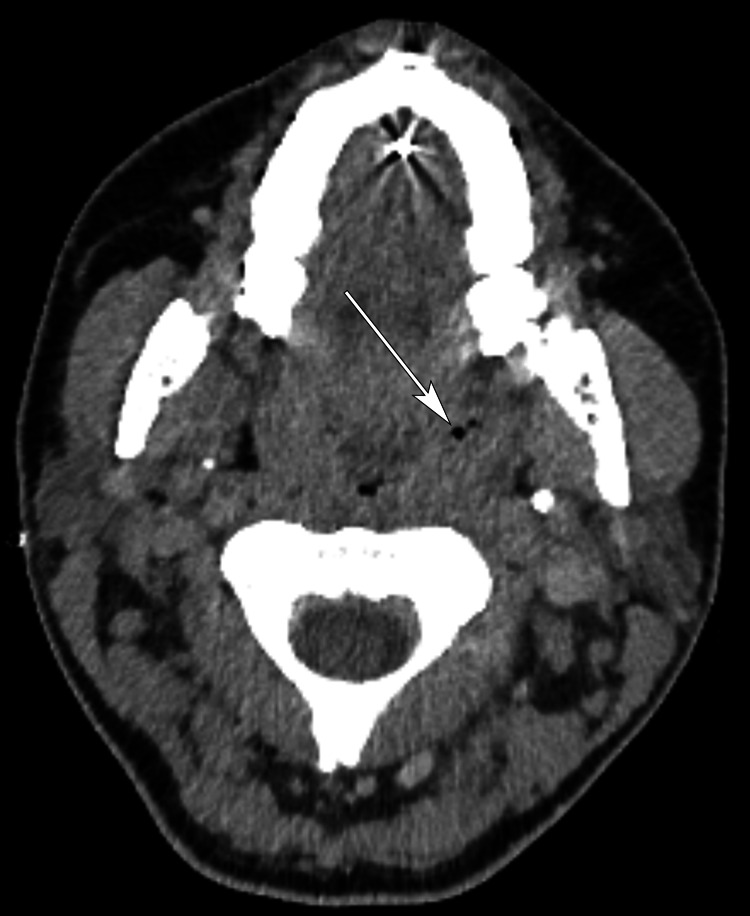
Postcontrast CT scan of the neck at the tongue base/tonsillar level There is symmetrical palatine tonsillar enlargement. No focal fluid collection is present, but bubbles of gas can be seen within the left palatine tonsil (arrow) consistent with infection with a gas-forming organism.
